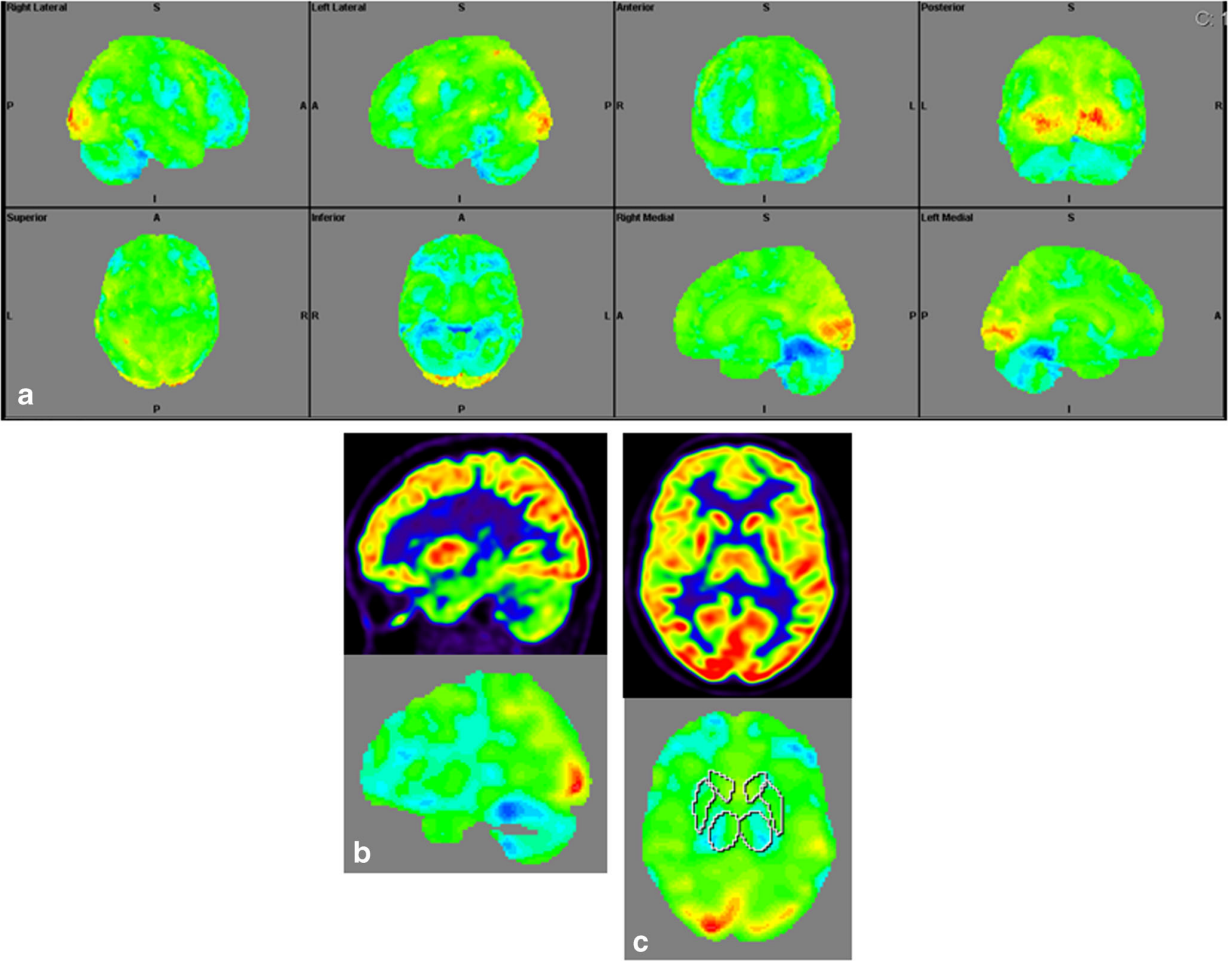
Surface projections (3DSSP) of brain [^18^F] FDG PET findings for patient IV:1. a Metabolism is reduced in the prefrontal and parietal cortex as well as in the entire cerebellum. b Midsagittal image shows reduced FDG uptake in the cerebellum (upper case) as compared to the reference values in the VOI templates (lower case). c A similar abnormality is evident in the thalami on the coronal image (upper case) as compared to the reference values (lower case), FDG uptake in the putamen is otherwise normal
